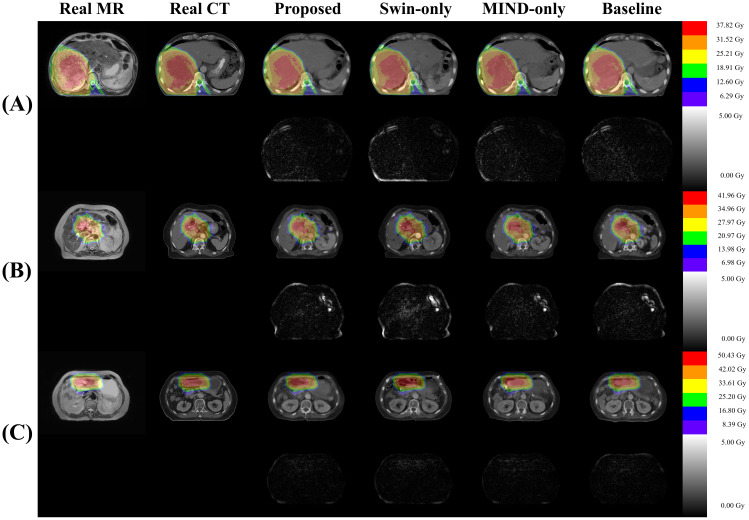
Comparison of dose distributions for magnetic resonance (MR), computed tomography (CT), and synthetic CT (sCT) images for three subjects (A–C). Each subpanel includes an overlay of dose distribution on the images in first row and the dose differences in the second row.
